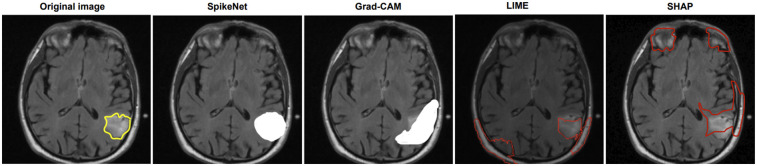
Visual comparison of explanation maps generated for a second representative brain MRI slice. The original image displays the expert-annotated tumour boundary (yellow), alongside explanation maps produced by SpikeNet (white), Grad-CAM (white), LIME (red), and SHAP (red).
